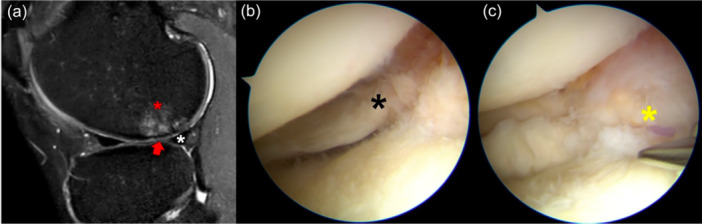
Right knee of a 33‐year‐old patient with previous isolated ACL reconstruction. The sagittal MRI of the lateral compartment shows the presence of subchondral bone oedema (red asterisk) and cartilage thinning, inhomogeneity, and abnormal signal (red arrow) at the lateral femoral condyle, with an intact lateral meniscus (white asterisk) (a). At the time of arthroscopy, the posterior root (black asterisk) was torn from the tibial insertion and floating (b). The root (yellow asterisk) was repaired with an all‐inside suture of the remnant (c). ACL, anterior cruciate ligament; MRI, magnetic resonance imaging.
